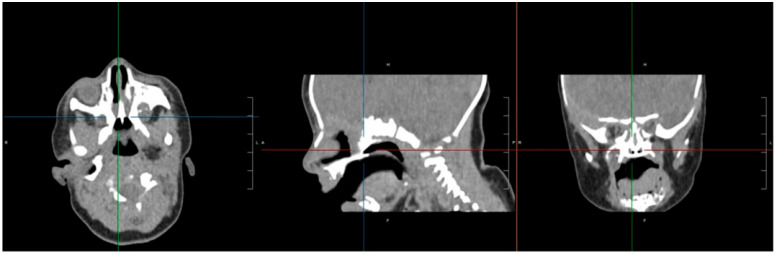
Skull CT highlights the presence of a bilateral membranous septum involving both choanae. The air column was interrupted on both sides; no bone changes were detectable, normal membranous appearance was due to incomplete physiological ossification of the planum ethmoidalis. There was no ethmoidal encephalocele. L: Left; R: right.
